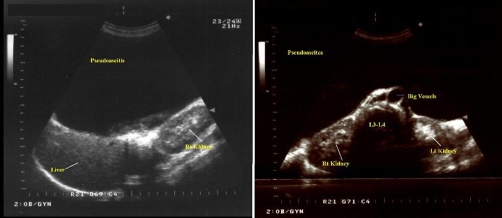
Transabdominal sonogram after paracentesis of the lesion. (Left) The liver and the right kidney are repelled to the posterior wall of the abdomen. The distance between the probe and the abdmominal organs is more than 13 cm. (Right) The large retroperitoneal vessels, the lumbar vertebrae and the two kidneys are seen.
